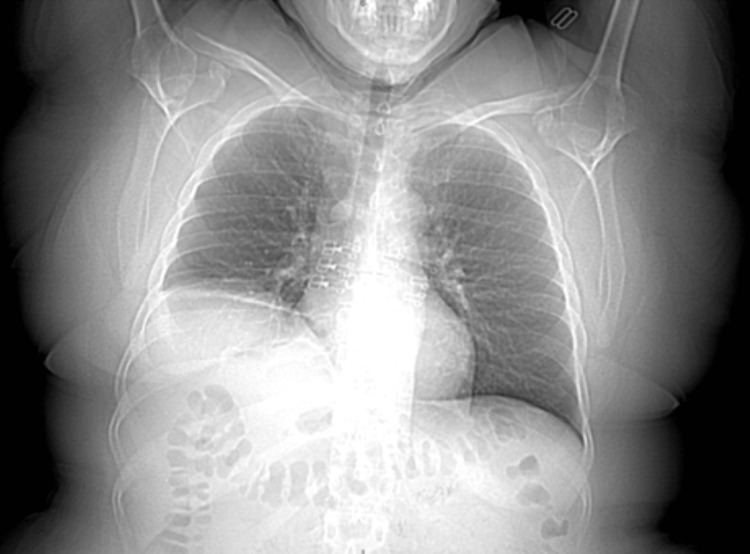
Coronal chest x-ray performed upon initial presentation to the ED showing right diaphragm paralysis.
